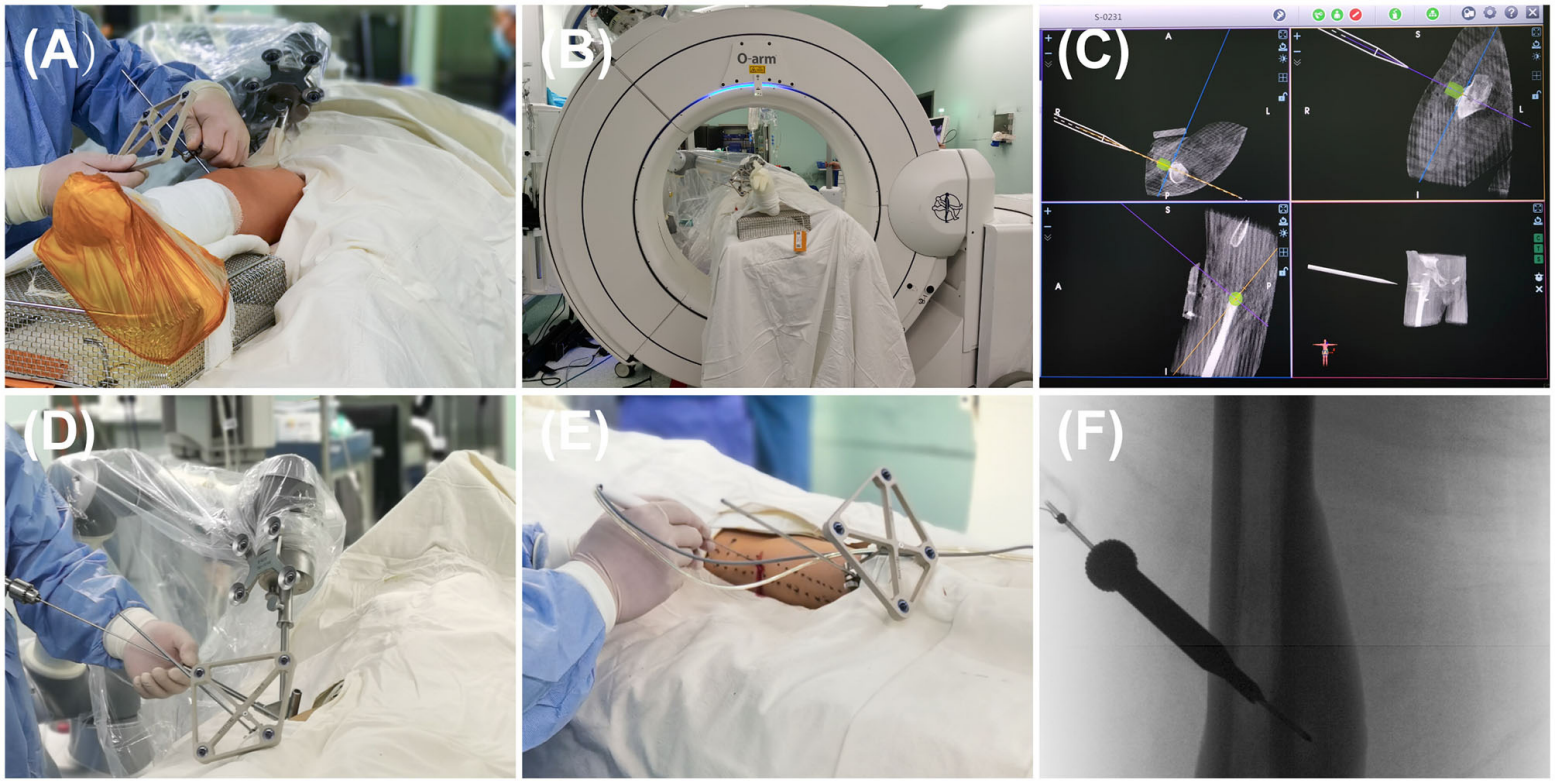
The process of robot-assisted, O-arm-navigated radiofrequency ablation (RFA) of osteoid osteoma (OO). (A) Tracer installation for the real-time capture of patient's spatial location. (B) Collecting the preoperative three-dimensional (3D) radiographs data and uploading it to the robot system. (C) 3D reconstructions and surgical path planning in the robot system. (D) The movement of robot's arm and director following the planned path, followed by the penetration of K-wire into the nidus with the assistance of robot's director. (E) The placement of RF needle into the nidus following the path of K-wire. (F) The radiographs of the RF needle localization.
